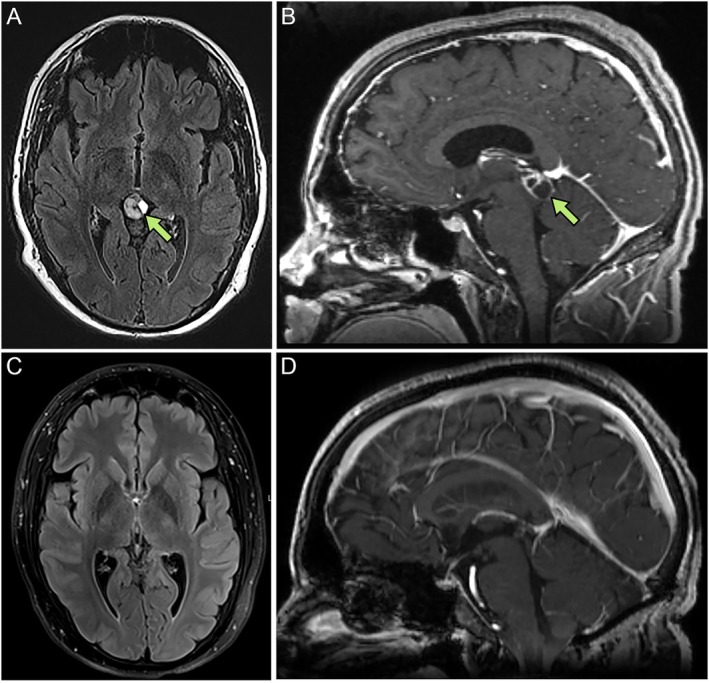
MRI of the initial pineal lesion and surveillance imaging after resection. (A) Axial T2‐weighted FLAIR image shows a lobulated, multicystic mass (arrow) centered in the pineal region without invasion of adjacent brain. (B) Sagittal postcontrast T1‐weighted image shows only thin peripheral enhancement without a solid or nodular enhancing component (arrow); the lesion has mild mass effect on the tectum with mild narrowing of the cerebral aqueduct but no hydrocephalus. Surveillance axial T2‐weighted FLAIR (C) and sagittal T1 post‐contrast (D) images at age 24 years (approximately 3 years after resection) show no evidence of recurrence.
